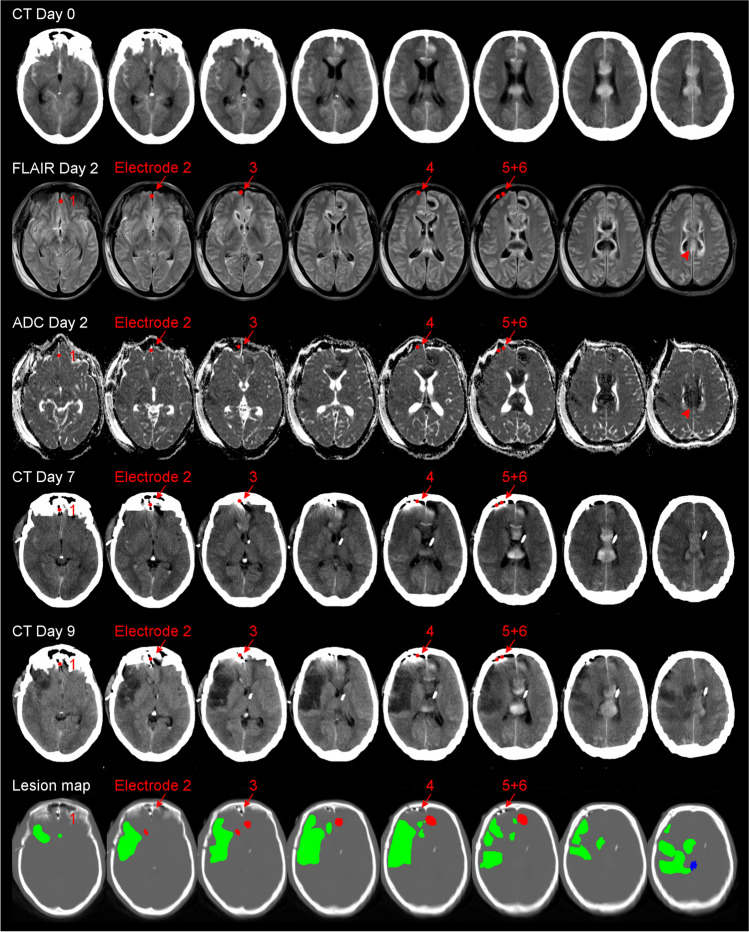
Development of a large delayed ischemic infarct between an MRI scan on Day 2 and two CT scans on Days 7 and 9 in a 46-year-old woman with aneurysmal subarachnoid hemorrhage (aSAH) due to rupture of an anterior communicating artery aneurysm. Representative CT and MRI slices in axial orientation. All images were aligned to the first postoperative T1 spin echo scan with a slice thickness of 5 mm. The initial CT scan on admission demonstrated subarachnoid blood in the basal cisterns and more superior in the callosal cistern and the interhemispheric fissure. Blood from the callosal cistern extended into the right frontal lobe and formed a small intracerebral hemorrhage (ICH) of 1 ml at a distance of 24 mm from subdural electrode 1. A small ICH with a volume of 4 ml was also found in the left medial frontal lobe originating from subarachnoid blood in the left cingulate sulcus. The postoperative MRI scan 52 h (Day 2) after onset of the initial hemorrhage showed symmetric, hyperintense bands in the cortex of the left and right superomedial margin on fluid attenuation inversion recovery (FLAIR) images with restricted diffusion on the apparent diffusion coefficient (ADC) map (see arrowheads). Furthermore, four hyperintense spots were found on diffusion-weighted imaging (DWI) in the right frontal and occipital cortex, the left frontal cortex, and the cerebellum (not shown). These findings are consistent with early brain infarction. Quantification yielded an infarct volume of 3 ml in the right hemisphere (shortest distance between infarct border and subdural electrode 5, 29 mm), 2 ml in the left hemisphere, and 1 ml in the cerebellum. Also note perihematomal edema surrounding ICH in both the right and left frontal lobe (hyperintense on FLAIR images, hypointense on the ADC map). On Day 7, CT revealed loss of gray-white matter differentiation in the right insular and frontal cortex. On Day 9, hypodensity and swelling became more marked with a midline shift of 6 mm and compression of the lateral ventricles. These findings are consistent with delayed cerebral infarction involving the vascular territories of the right middle cerebral artery (MCA) and anterior cerebral artery (ACA). Volume analysis revealed a tissue loss of 107 ml due to delayed cerebral ischemia (DCI) ipsilateral to the subdural electrodes. The lowest row presents the CT scan (bone window) on Day 9. The subdural electrode strip is visible over the medial and lateral surface of the right frontal lobe (electrodes are marked with red arrows). Additionally, the quantified lesions from the scans above are projected onto the CT images. The red-labeled area indicates ICH, the blue area early infarction in the MRI on Day 2, and the green area delayed infarction in the CT on Day 9. Note the proximity between the delayed ischemic infarct and electrode 6, which was at 6 mm from the infarct
